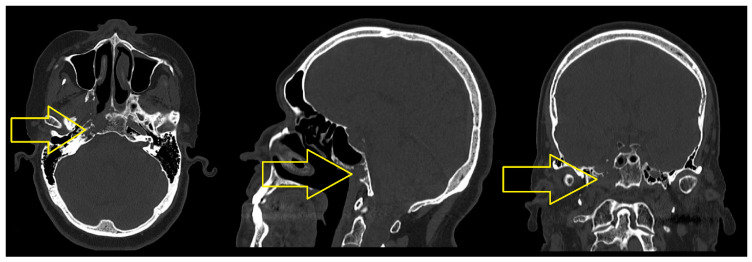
The computed tomography of the cervical area with contrast medium—October 2019. The nasopharyngeal tumor with osteolysis at the base of the cranium (yellow arrow), with extension of 30/20/15 mm.
